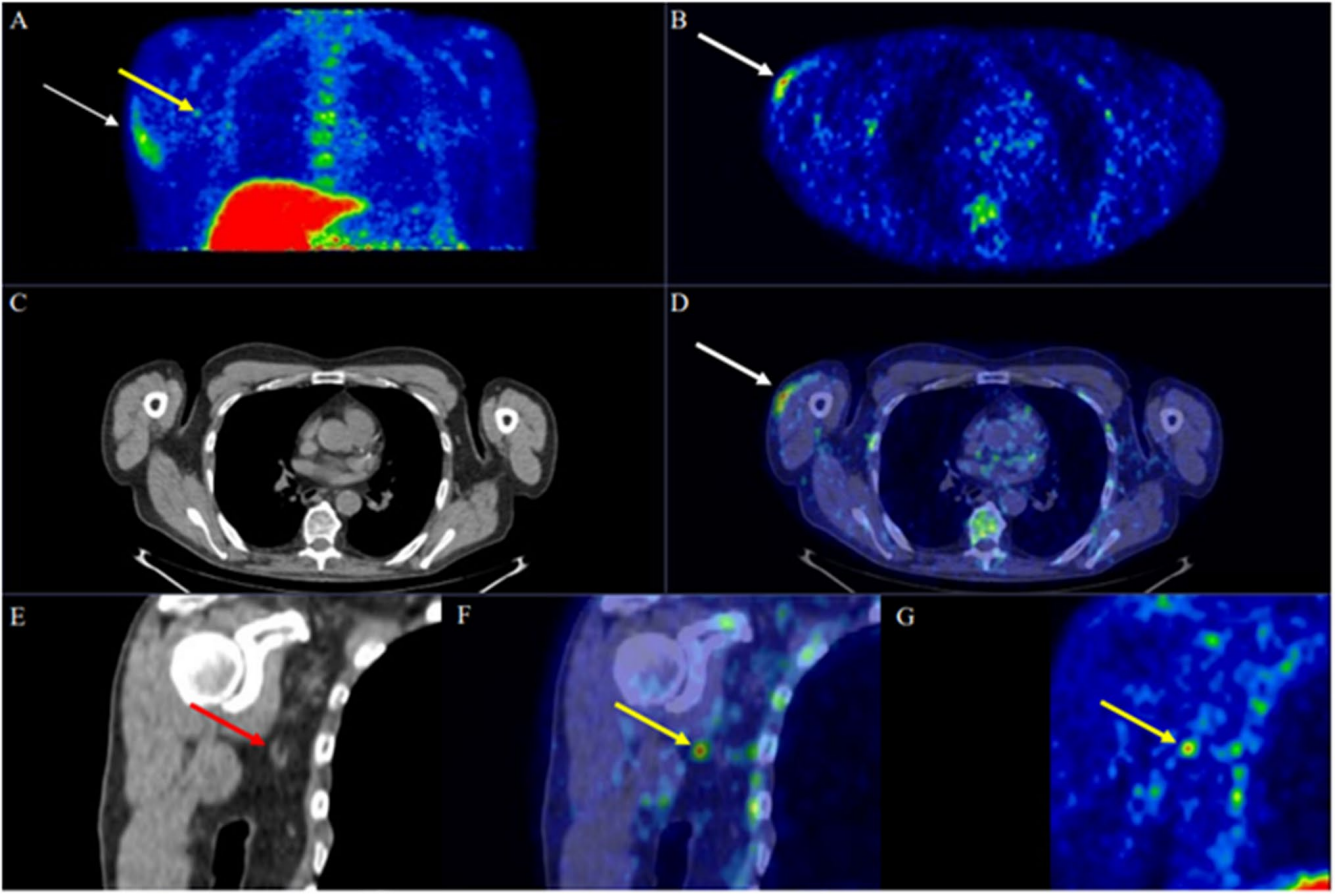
[^18^F]Florbetaben PET/CT: MIP (A), PET (axial-B, coronal-G), CT (axial-C, coronal-E), PET/CT (axial-D, coronal-F) images demonstrated ill-defined uptake in the right arm’s subcutaneous tissues (SUVmax 5.6; white-arrows) and next to a possible right-axillar lymph node (SUVmax 4.75; yellow-arrows) evident on low-dose CT scan without breathing control (red arrows). Reprinted with permission from Nuclear Medicine Unit, Fondazione Istituto G. Giglio, Cefalù (Palermo), Italy.
